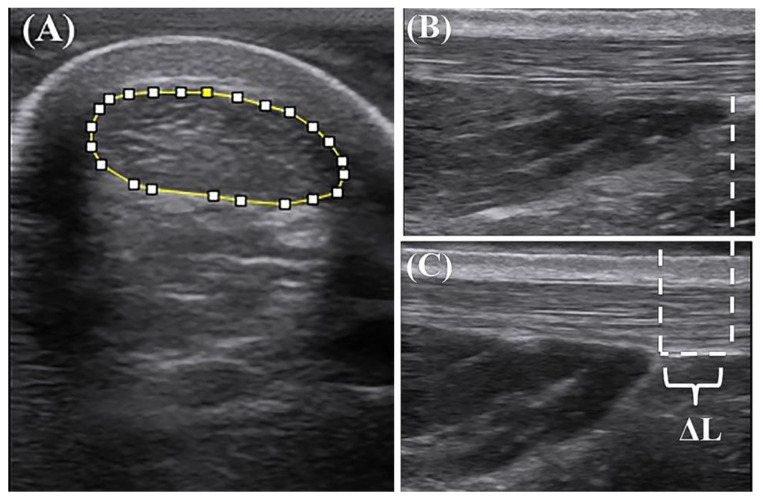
(A) Ultrasound image of the cross-sectional area; (B) Achilles-soleus myotendinous junction at rest; (C) Achilles-soleus myotendinous junction at 100% maximum voluntary isometric contraction. ΔL, the change in the length of the proximal Achilles tendon during the maximum plantar flexion isometric contraction.
